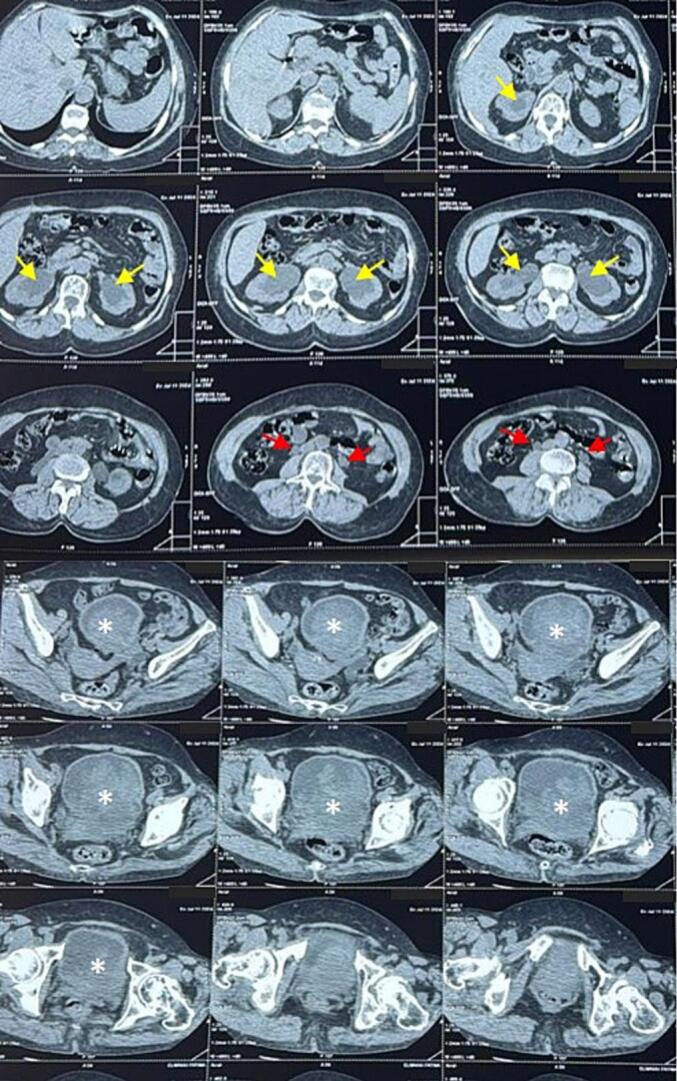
Non-contrast CT scan: Bilateral ureterohydronephrosis with symmetrical pyelocaliceal (yellow arrow) and ureteral dilation (red arrow) upstream of a 4 cm trigonal bladder lesion (white asterisk). (For interpretation of the references to colour in this figure legend, the reader is referred to the web version of this article.)
